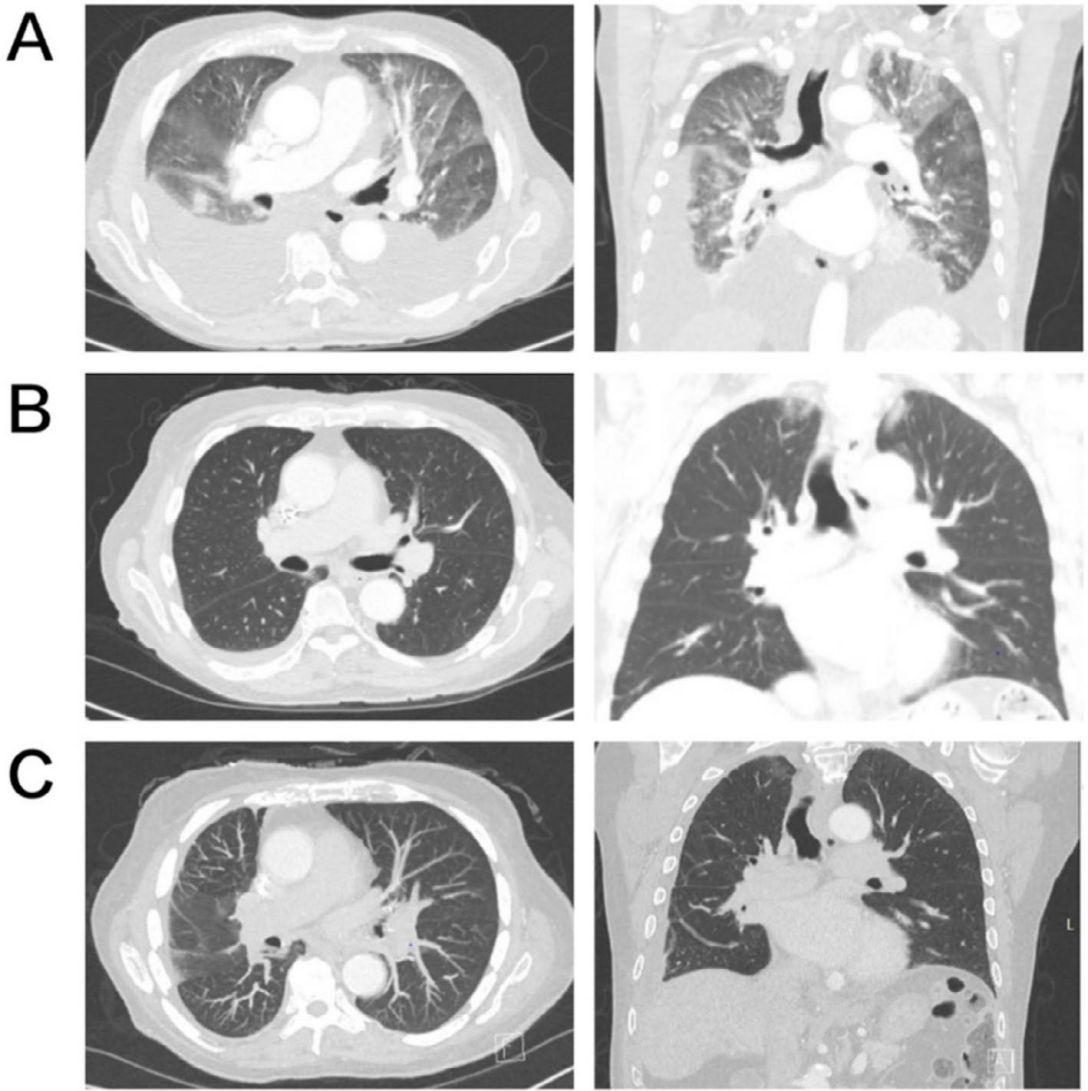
Radiographic response to olaparib on CT scans. (A) CT chest with contrast before olaparib revealed bilateral consolidative opacities in the left apex, right middle lobe, and right lower lobe, together with moderate‐to‐large pleural effusions. (B) Surveillance CT obtained 7 months after initiating olaparib demonstrated significant interval decrease in pleural effusions and resolution of the areas of consolidation within the left apex, right middle lobe, and right lower lobe. (C) Eighteen‐months follow‐up CT showed further diminution of the residual right pleural effusion, with no evidence of recurrent parenchymal disease.
